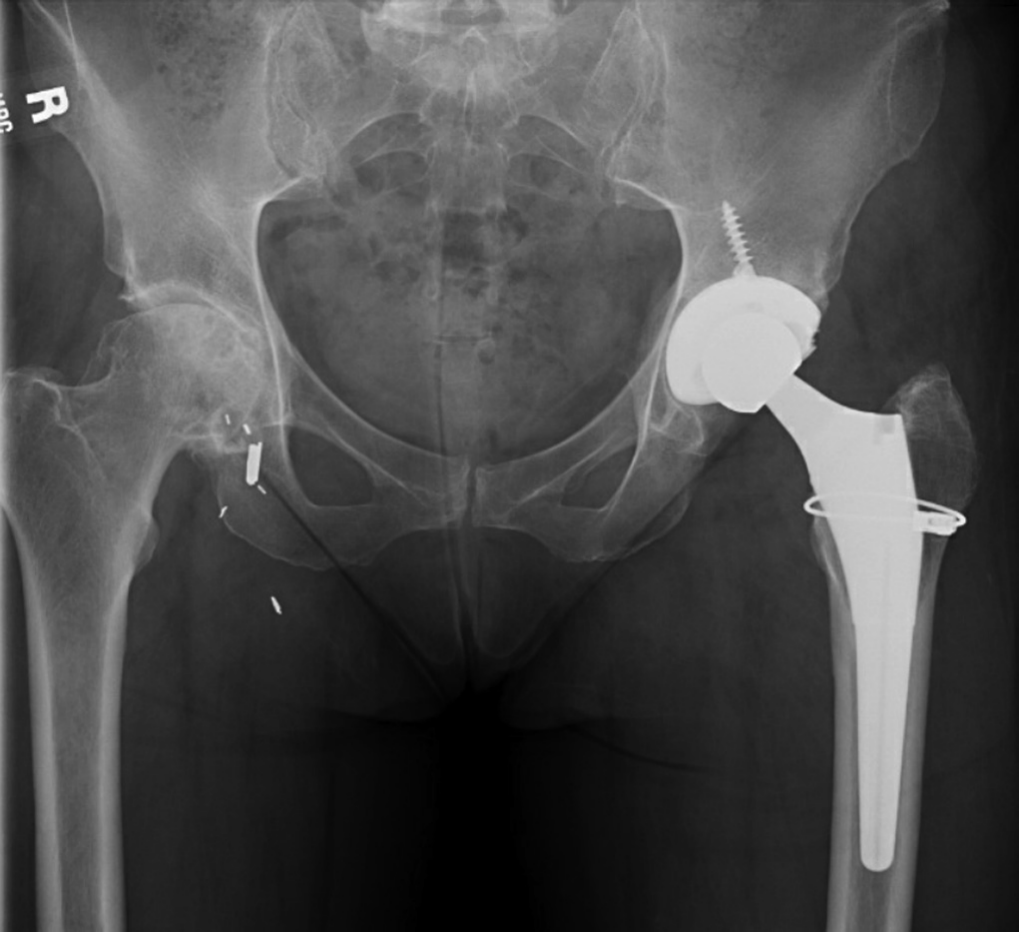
Pelvic radiograph 2 years after first-stage L-THA and 7 months before R-THA, October 2012.
